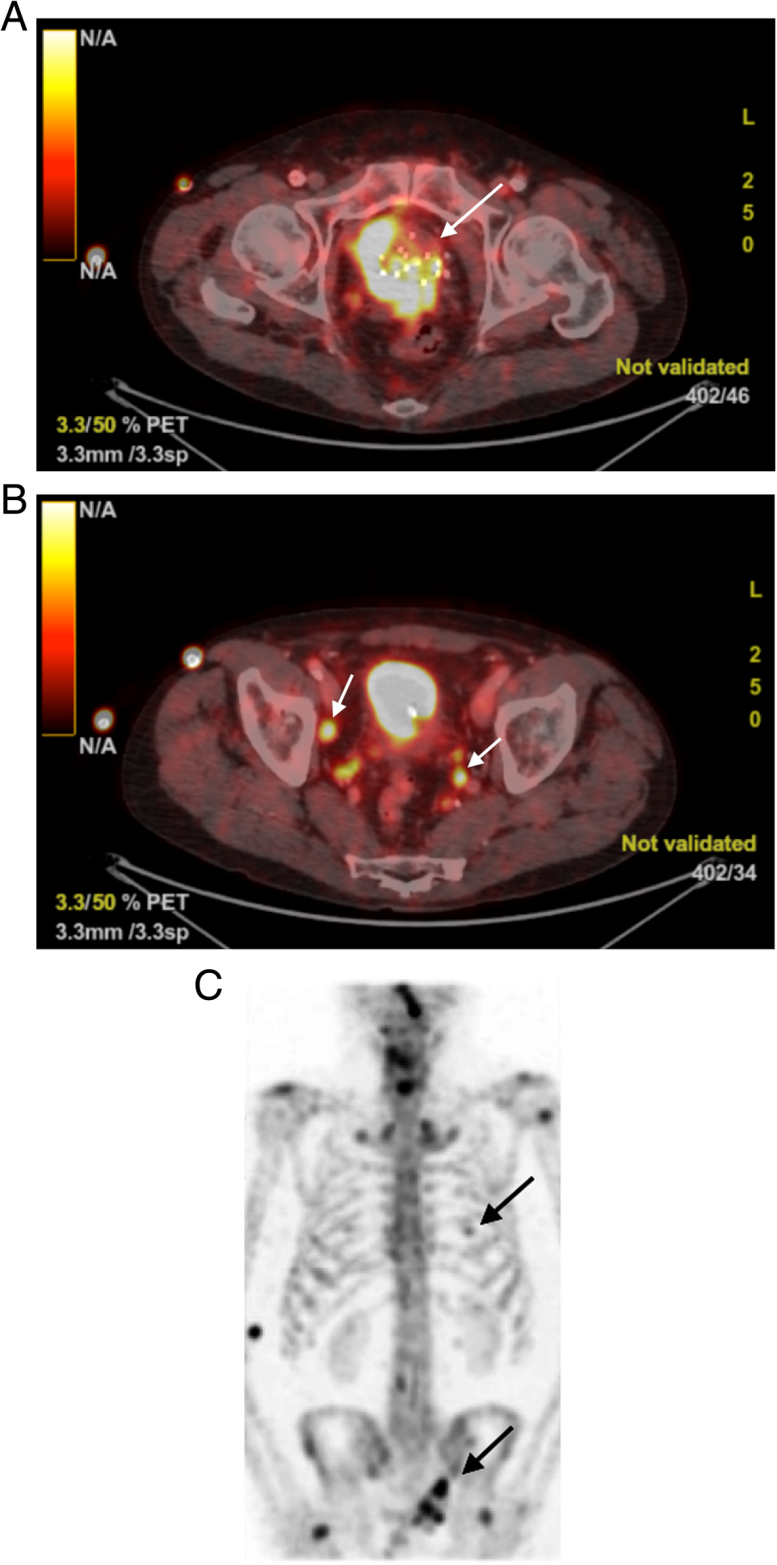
a Tumoral prostate with invasion of the posterior bladder wall. The arrow points to the brachytherapy seeds in the prostate. b Right arrow shows positive lymph nodes in the iliac region and left arrow in the obturator region. c Upper arrow points to osteoblastic lesions in the rib, lower arrow in the sacrum and left os ileum on bone scintigraphy
